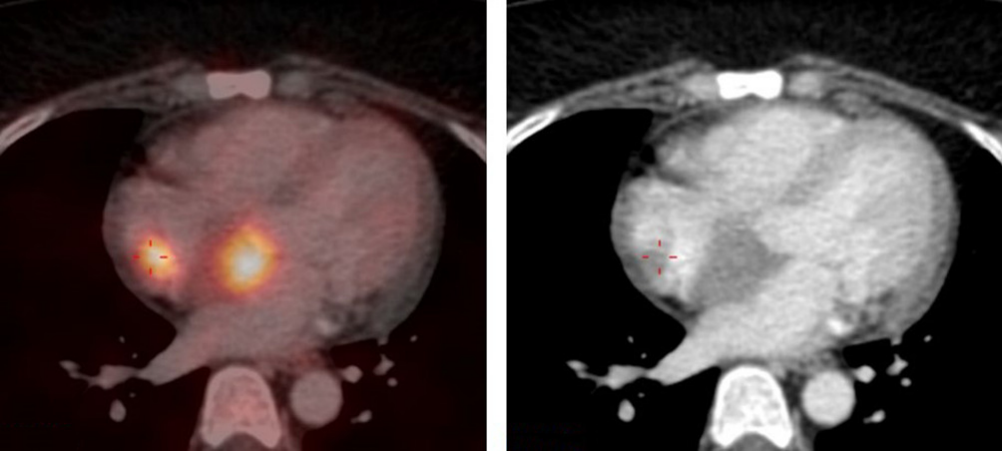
Left: ^18^F-fludeoxyglucose uptake in wall of right atrium and left atrium. Right: CT image showing deposits in the walls of the right and left atrium
